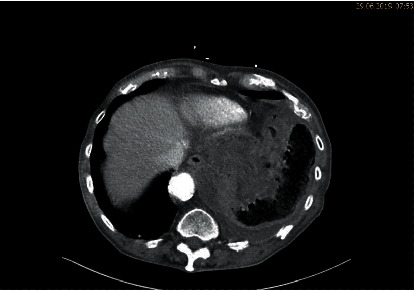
Axial thoracoabdominal CT scan with left-sided seropneumothorax.
